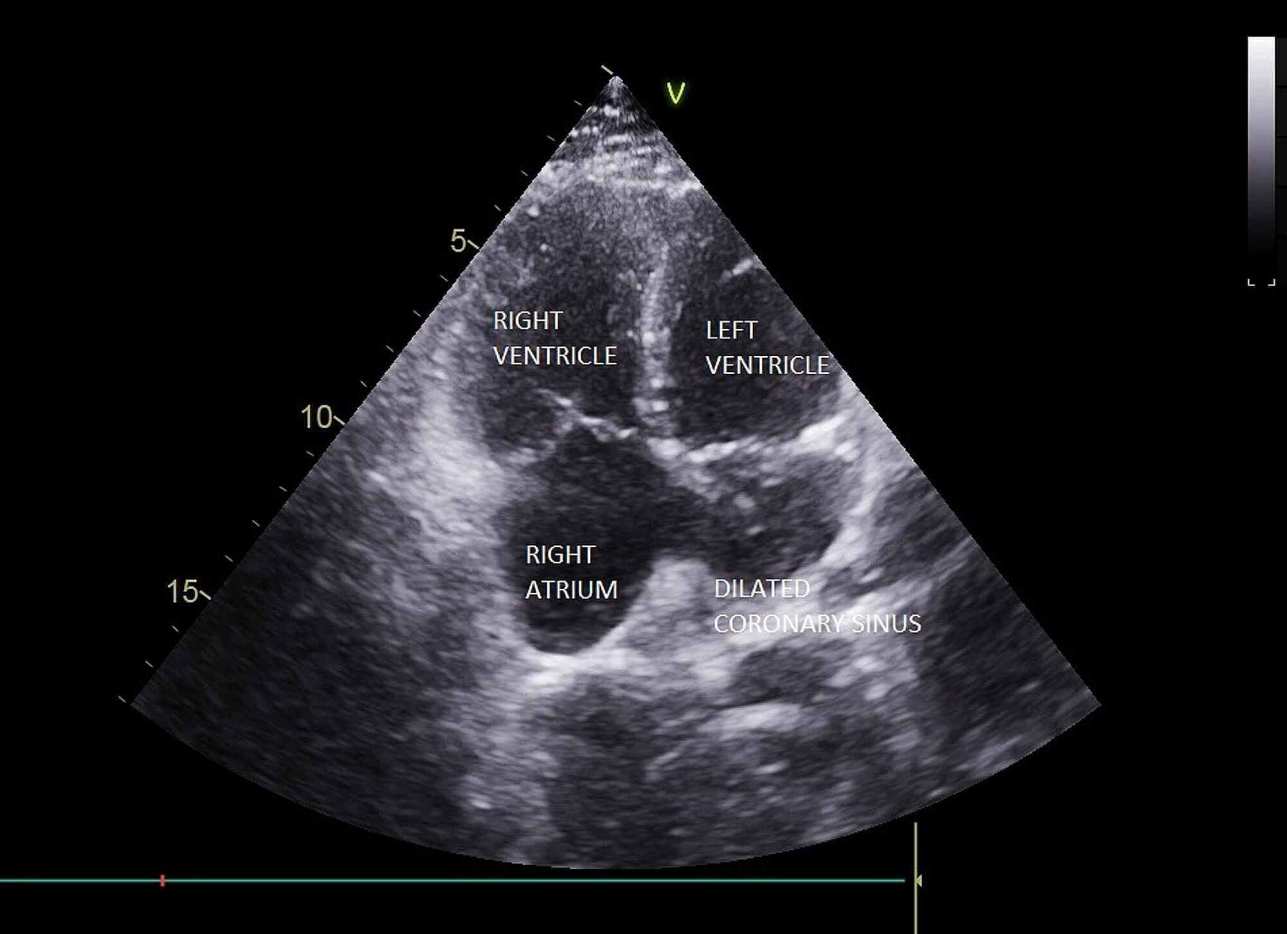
Foreshortened apical four-chamber view showing a dilated coronary sinus
